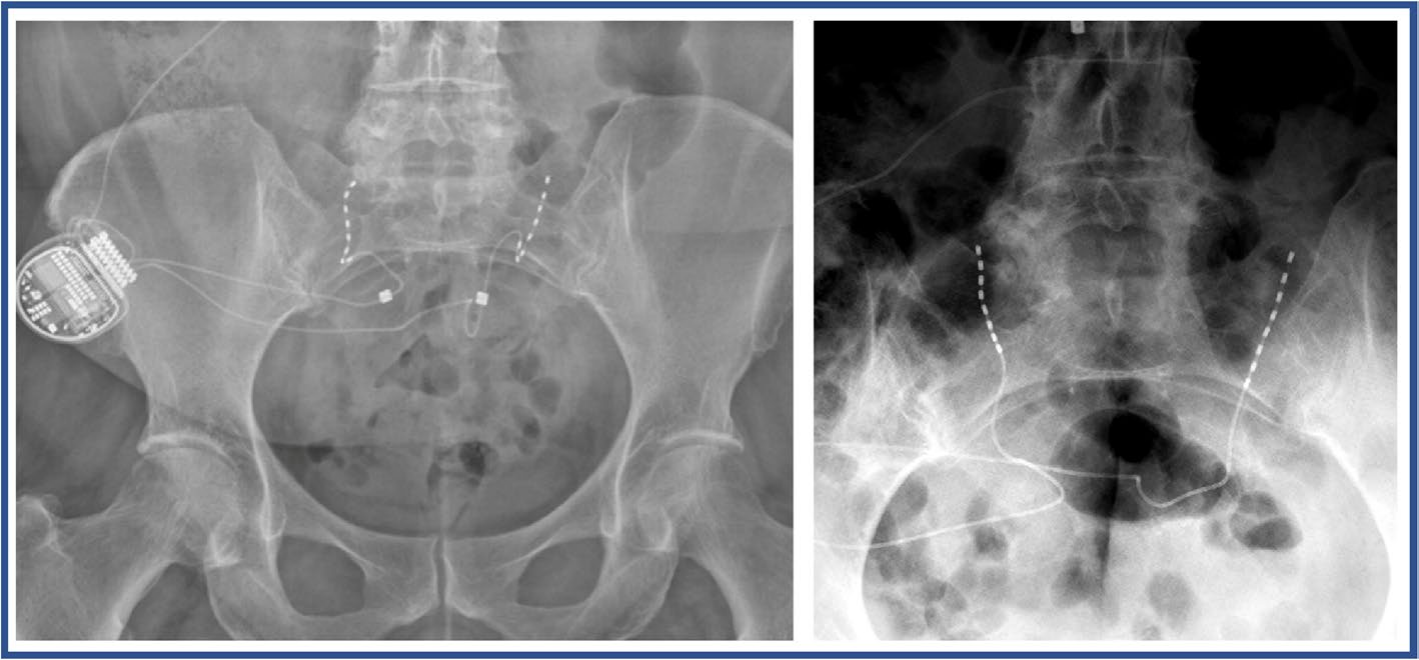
Postoperative coronar X-rays of two different patients with placement of the leads between the sacroiliac joint and the sacral foramina. Note that the Implantable Pulse Generator in the gluteal pocket is displayed on the right
